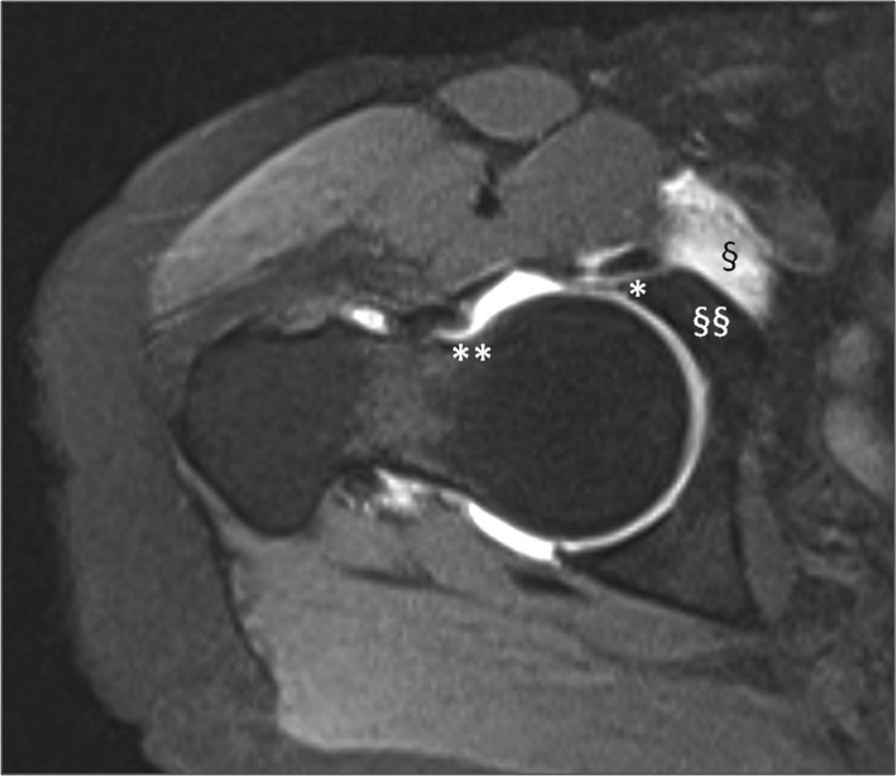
Femur-based pincer FAI morphology. This arthro-MRI axial reconstruction performed with neutral rotation of the limb shows a retroverted neck of femur and some particular features of pincer FAI (*degenerated labrum; ^^indentation line; ^§^ilio-pectineal *bursa* dilated by contrast medium; ^§§^no signs of cartilage delamination)
